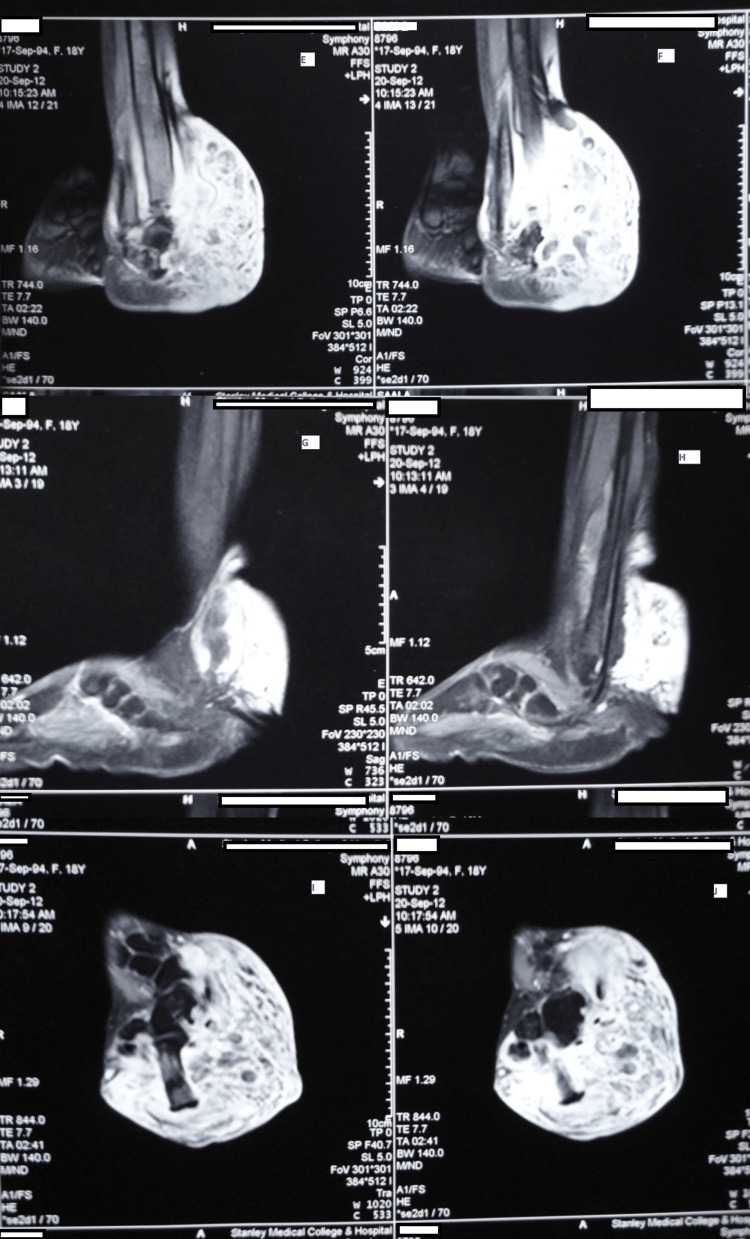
MRI of the right ankle swelling was described as T1 hypointense and T2 hyperintense lesion in the posterolateral aspect of the ankle.
